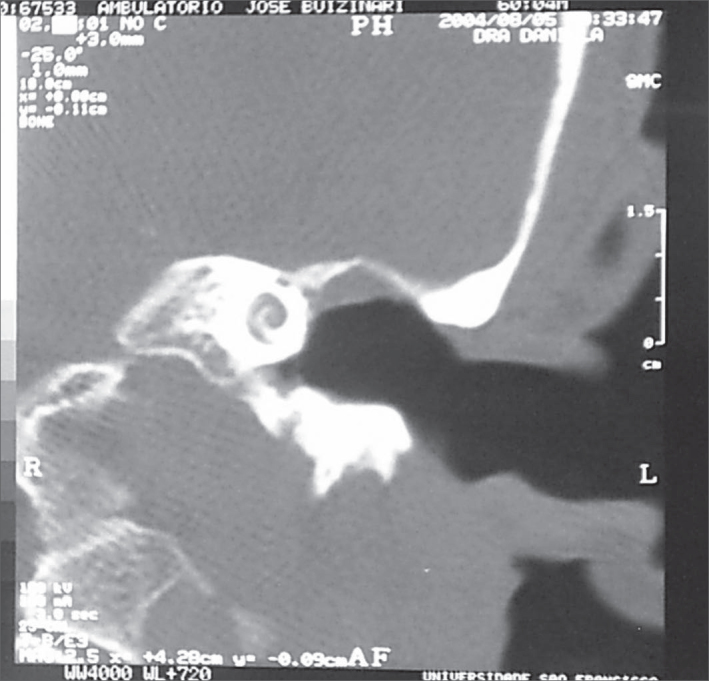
Computed tomography, coronal section, showing radical mastoidectomy cavity to the left.
